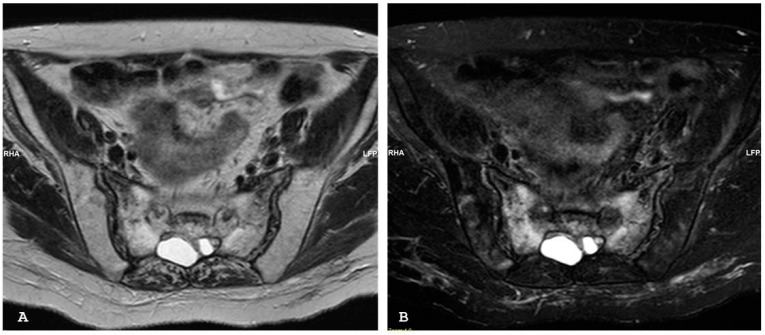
Axial T2-weighted (A) and axial short tau inversion recovery (B) magnetic resonance imaging sequences show hypointense fracture lines with surrounding acute bone marrow edema within the sacrum. Note the sacral Tarlov perineurial cysts.
